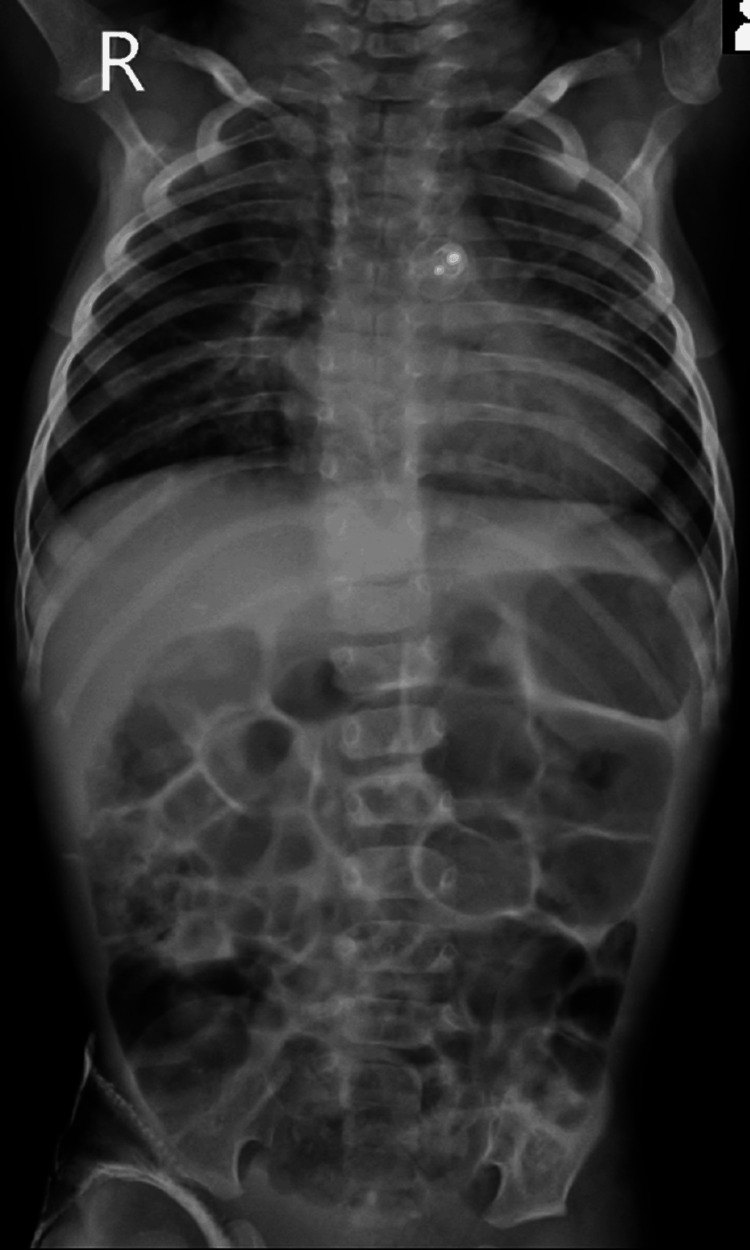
Post-operative chest radiograph showing bilaterally clear lung fields with amplatzer PDA device in situ. Significant improvement was seen symptomatically as well as in the chest radiograph post surgery and mechanical ventilation.
